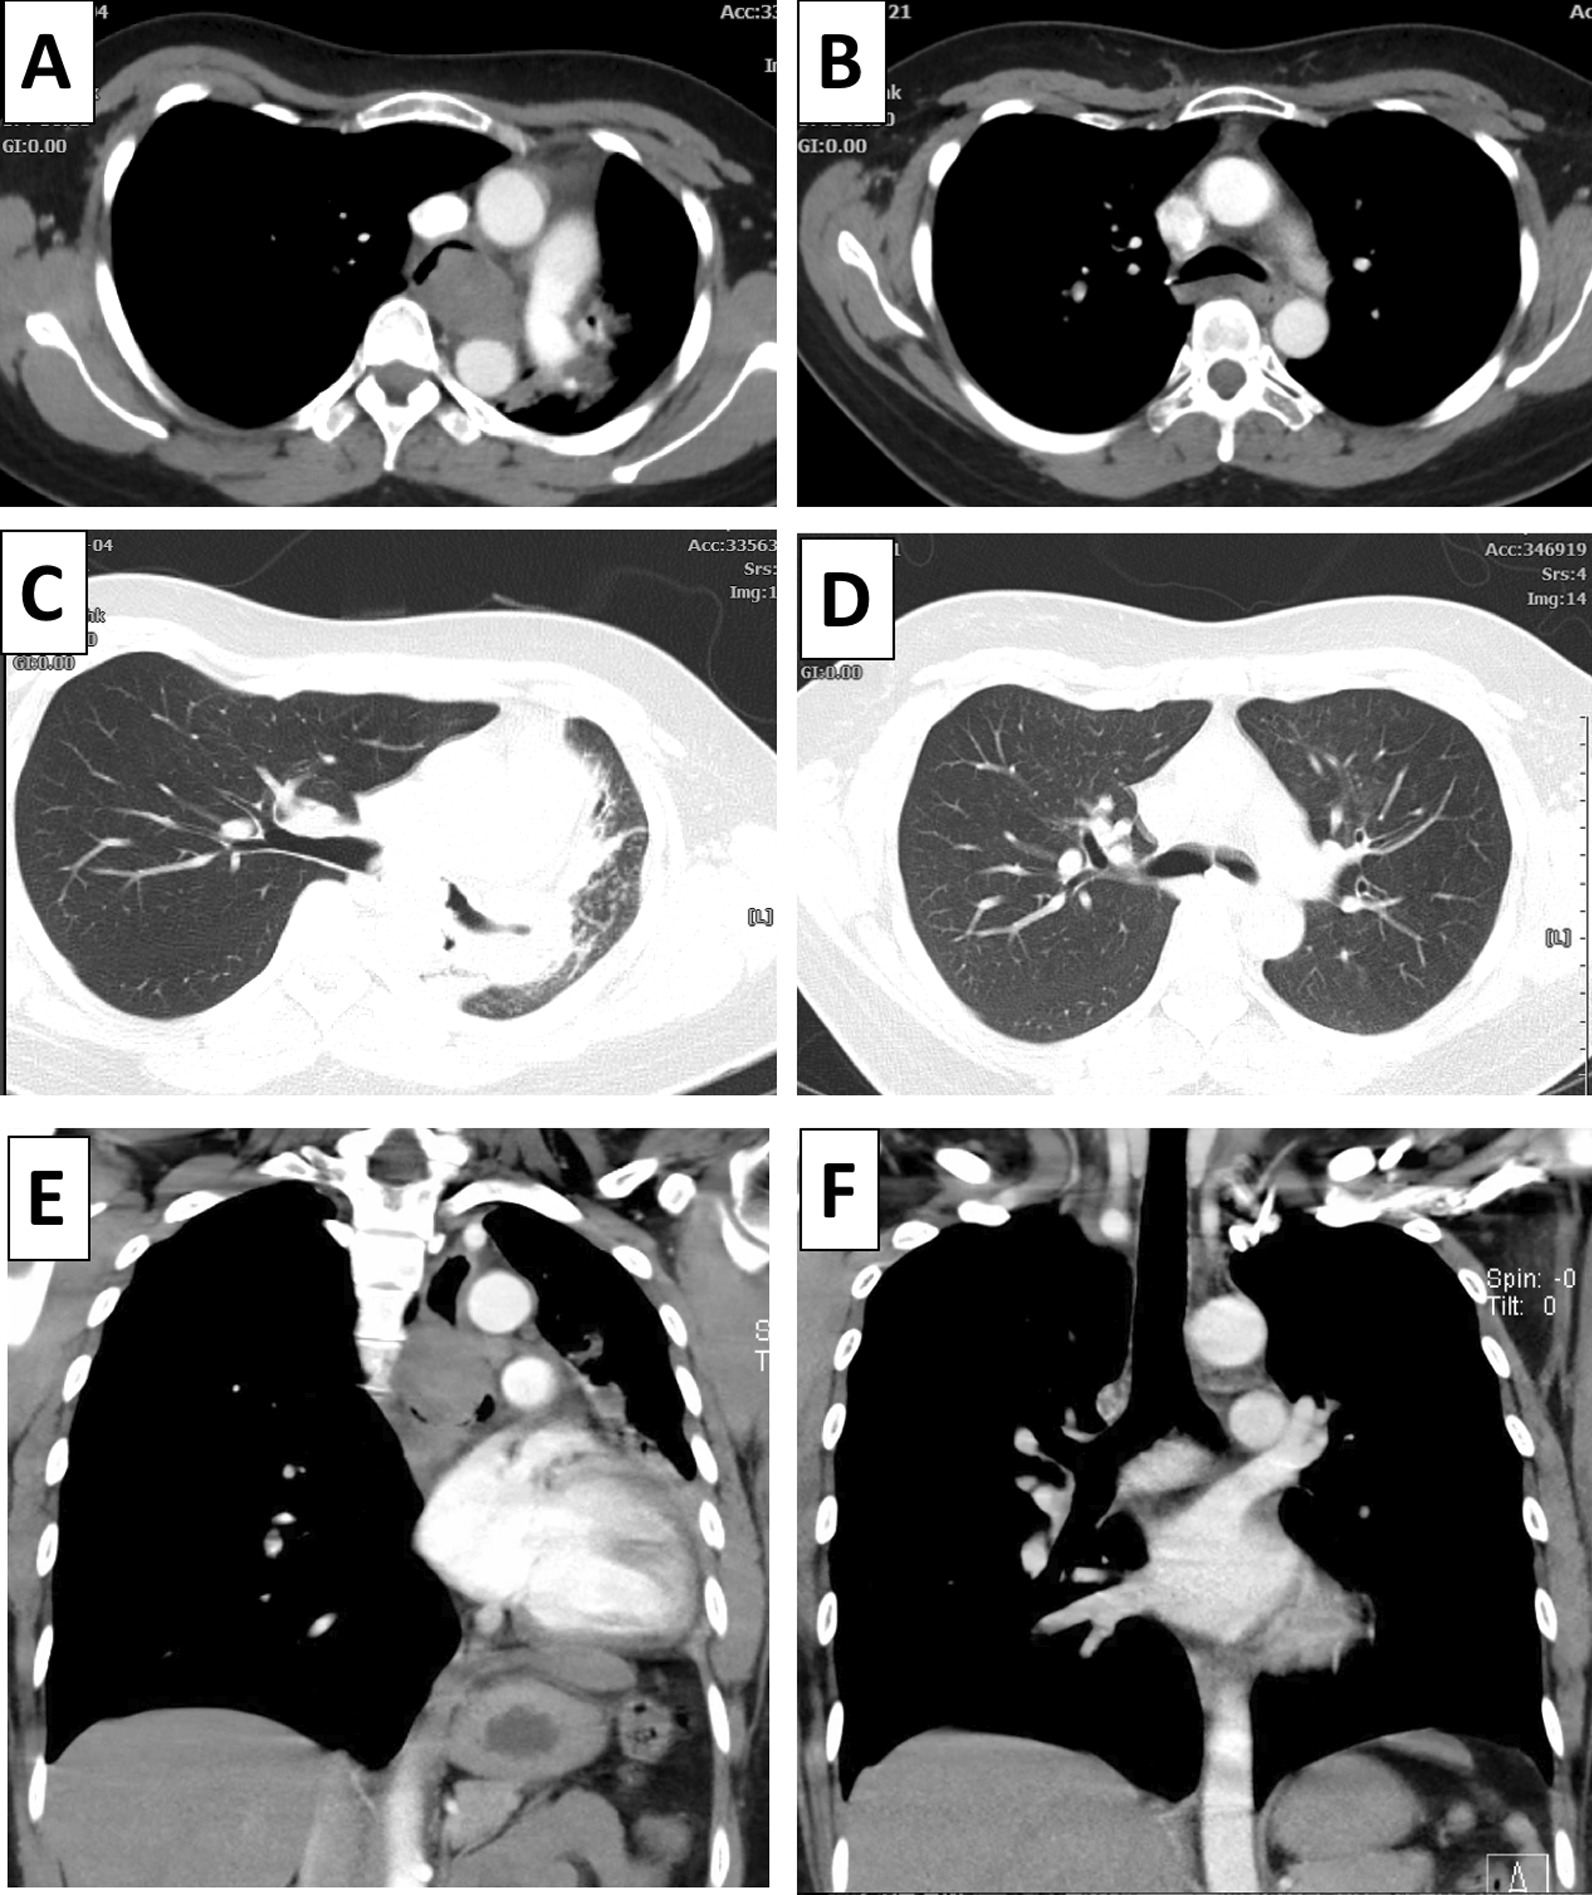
Thoracic computed tomography (CT) scan before and after treatment. A Axial mediastinal window of the chest CT scan taken before the procedure, showing a mass on the left main bronchus. B Axial mediastinal window of the chest CT scan taken 2 months after radiotherapy and chemotherapy, showing no visible mass. C Axial lung window of the CT scan taken before the procedure, showing segmental atelectasis of the left lung. D Axial lung window of the chest CT scan taken 2 months after radiotherapy and chemotherapy, showing no pulmonary mass. E Coronal mediastinal window of the chest CT scan taken before the procedure, showing a mass on the left main bronchus. F Coronal mediastinal window of the chest CT scan taken 2 months after radiotherapy and chemotherapy, showing no pulmonary mass
